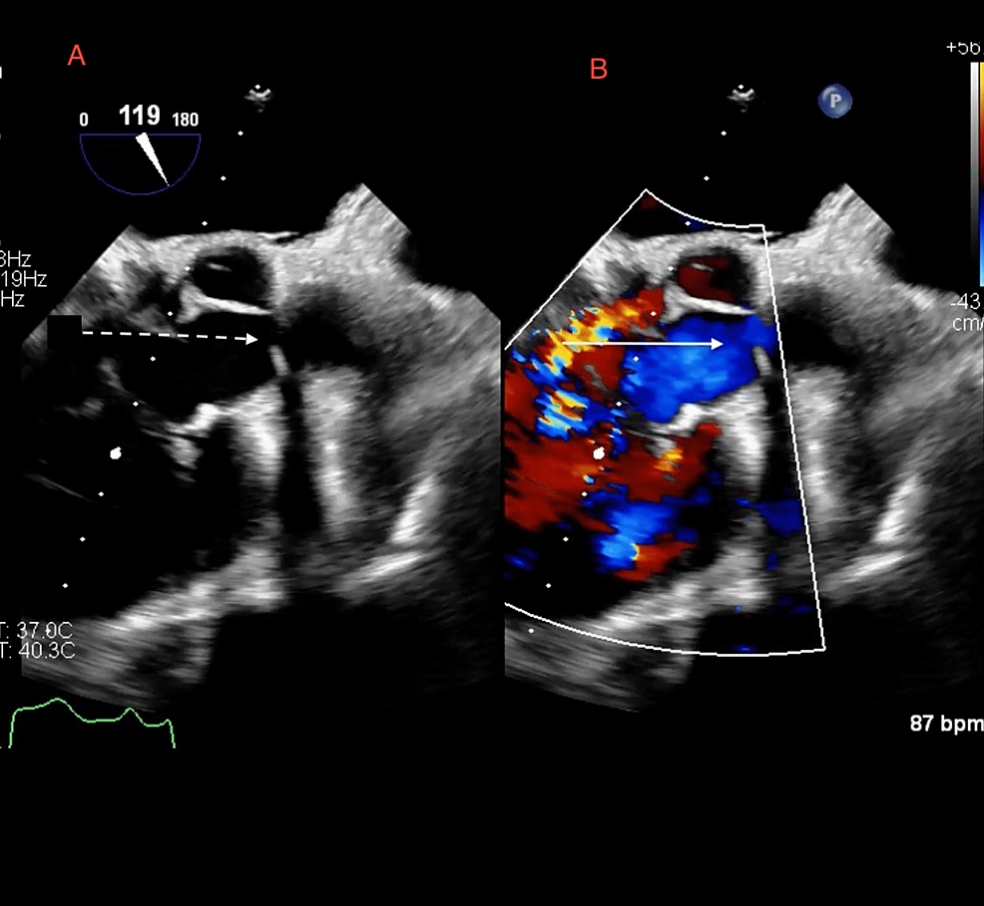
TEE images A: Visualization of the right SVA (dotted arrow),  B: Color Doppler showing intra-cardiac shunting at the site of SVA rupture (straight arrow) TEE: Transesophageal echocardiogram, SVA: Sinus of Valsalva aneurysm
